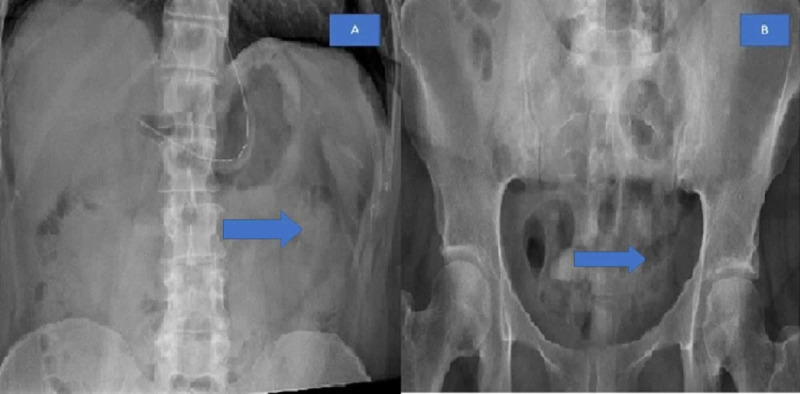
X-ray 4A, 4B: Repeat X-ray abdomen in 24 hours shows complete resolution of bowel obstruction and normal gas pattern (shown by arrows)
